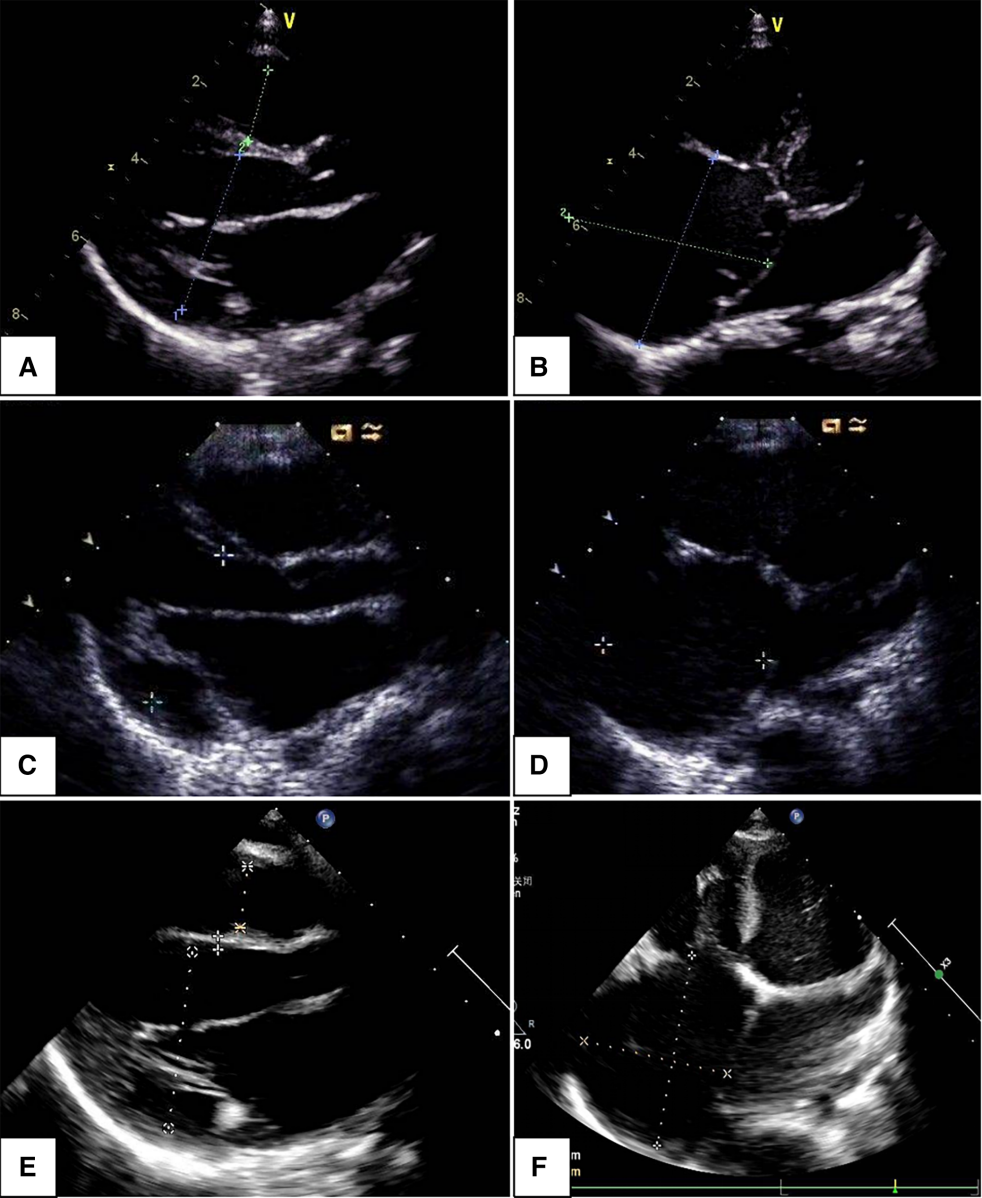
Changes in echocardiogram with follow-up time: parasternal long axis view indicated left ventricular end-diastolic dimension increased within 1, 2, and 5 years (A,C,E); apical four-chamber view showed an enlarged right atrium, the changes were not significant within 1, 2, and 5 years (B,D,F).
